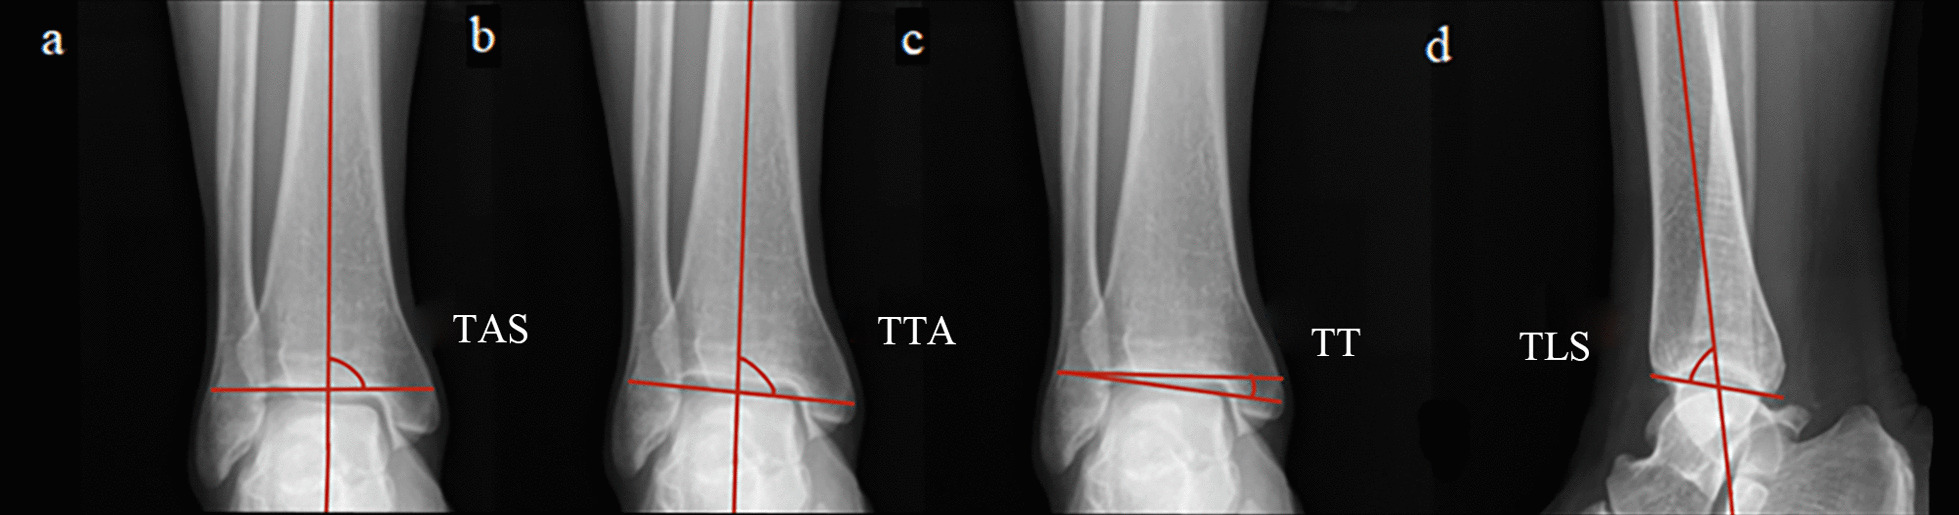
The angle is mainly measured by the ankle weight-bearing radiographs. a TAS: Tibial articular surface angle; b TTA: talar tilt angle; c TT: tibiotalar surface angle; d TLS: tibial lateral surface angle
